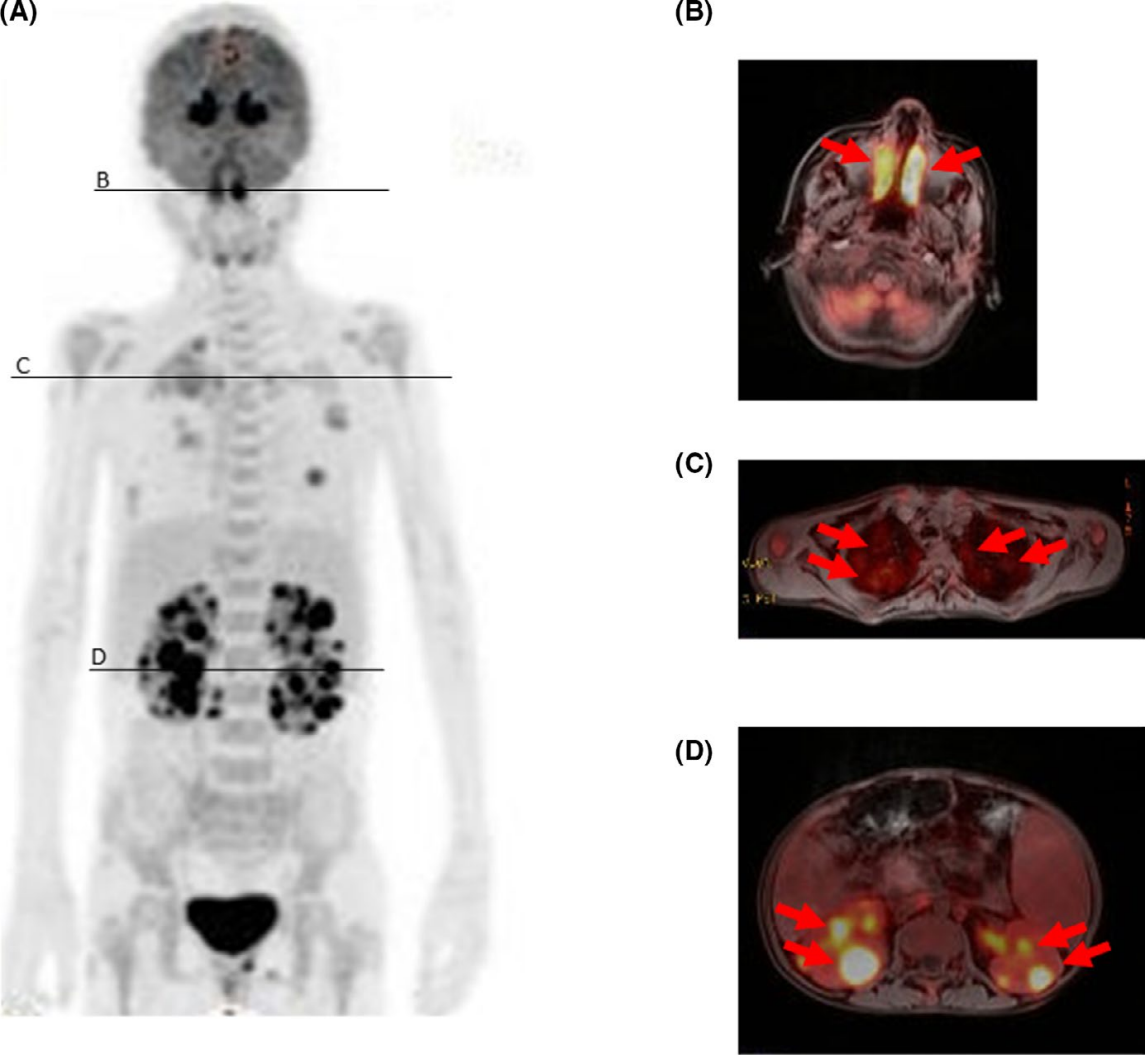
PET‐MRI images. A, Whole scan showed abnormal accumulations in the sinusoid, lungs, and kidney. B‐D, Coronal images of each organ. B, sinusoid; C. lungs; D, kidney
